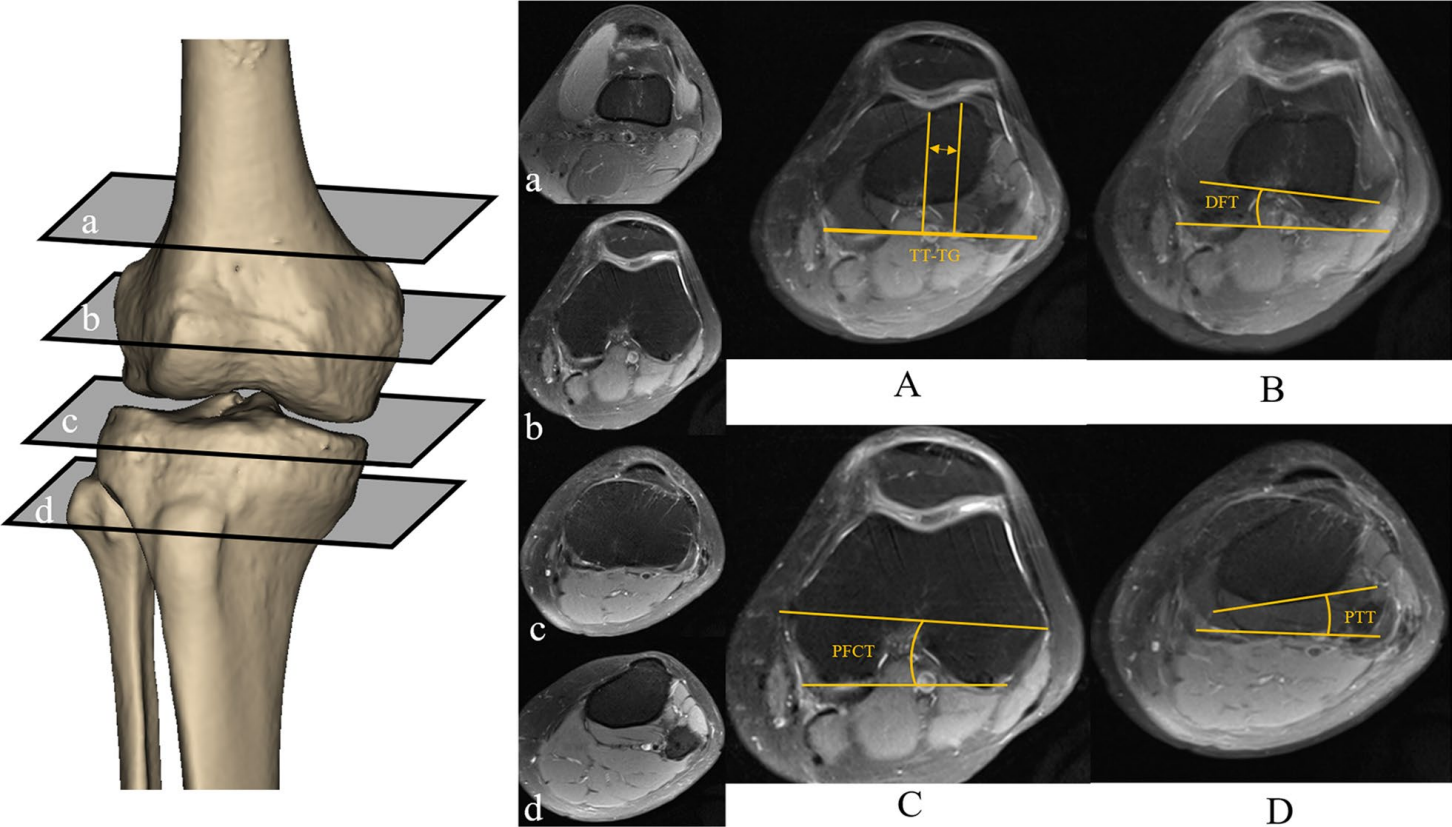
Measurements of TT-TG, DFT, PFCT and PTT. a, Slice above the gastrocnemius insertion of the distal femur. b, Slice of the gastrocnemius insertion of the distal femur. c, Slice of the proximal tibia. d, Slice of the level of the tibial tuberosity. A The TT-TG distance is the superposition of slices b and d. The TT-TG distance is defined as the distance measured on MRI between the most anterior part of the tibial trochanter and the middle of the trochlear groove. B The DFT is the superposition of slices a and b. The DFT is defined as the angle between the posterior cortical line of the distal femur and the posterior condylar line. C PFCT is defined as the angle between the line passing through the medial sulcus/apex of lateral epicondyle and the line passing through the posterior condylar axis on the slice b. D The PTT is the superposition of slices c and d. The PTT is defined as the angle between the line that joins the posterior border of the tibial plateaus at the level of the tibial insertion of the posterior cruciate ligament and the line that joins the posterior border of the tibia at the level of the tibial tubercle. TT-TG, tibial tubercle to trochlear groove. DFT, distal femoral torsion. PFCT, posterior femoral condylar torsion. PTT, proximal tibial torsion
